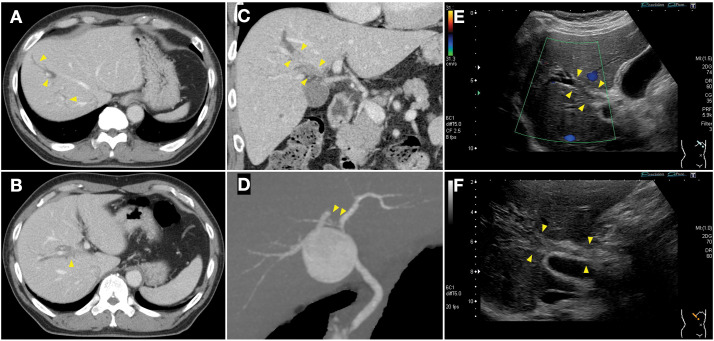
Preoperative imaging studies. (A–C) Contrast-enhanced CT (CECT). The intrahepatic bile ducts of the anterior section are dilated (A), and solid components fill the right hepatic duct and bile duct at the origin of segment B8 (B). Coronal reconstructed CECT clearly showing the intraductal lesion and upstream bile duct dilatation (C). (D) Drip infusion cholangiographic CT. A filling defect can be observed in the right hepatic duct; however, the bile ducts in the anterior section are not visualized. (E, F) Abdominal ultrasonography. Solid components can also be observed within the right hepatic and bile ducts at the origin of B8, with peripheral intrahepatic bile duct dilation. Arrowheads indicate the location of the intraductal lesion in all panels.
